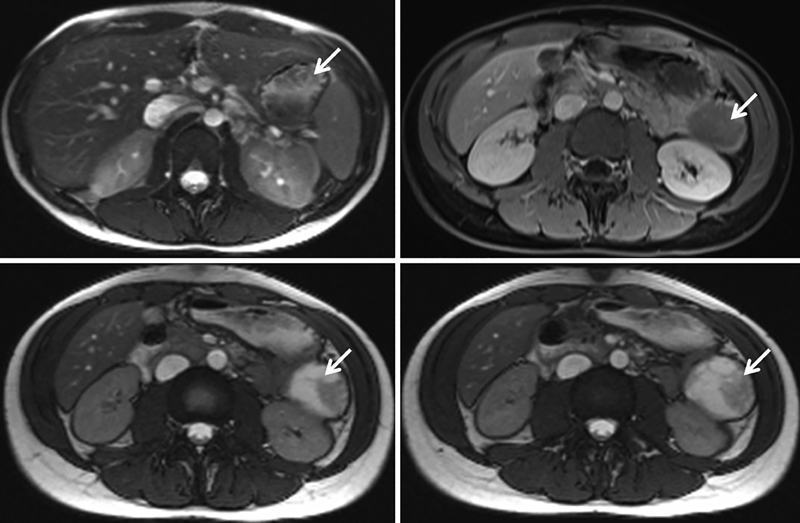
Axial contrast-enhanced MRI of the abdomen showing the tumorous lesion (white arrow) and its relation to adjacent organs. MRI, magnetic resonance imaging.
